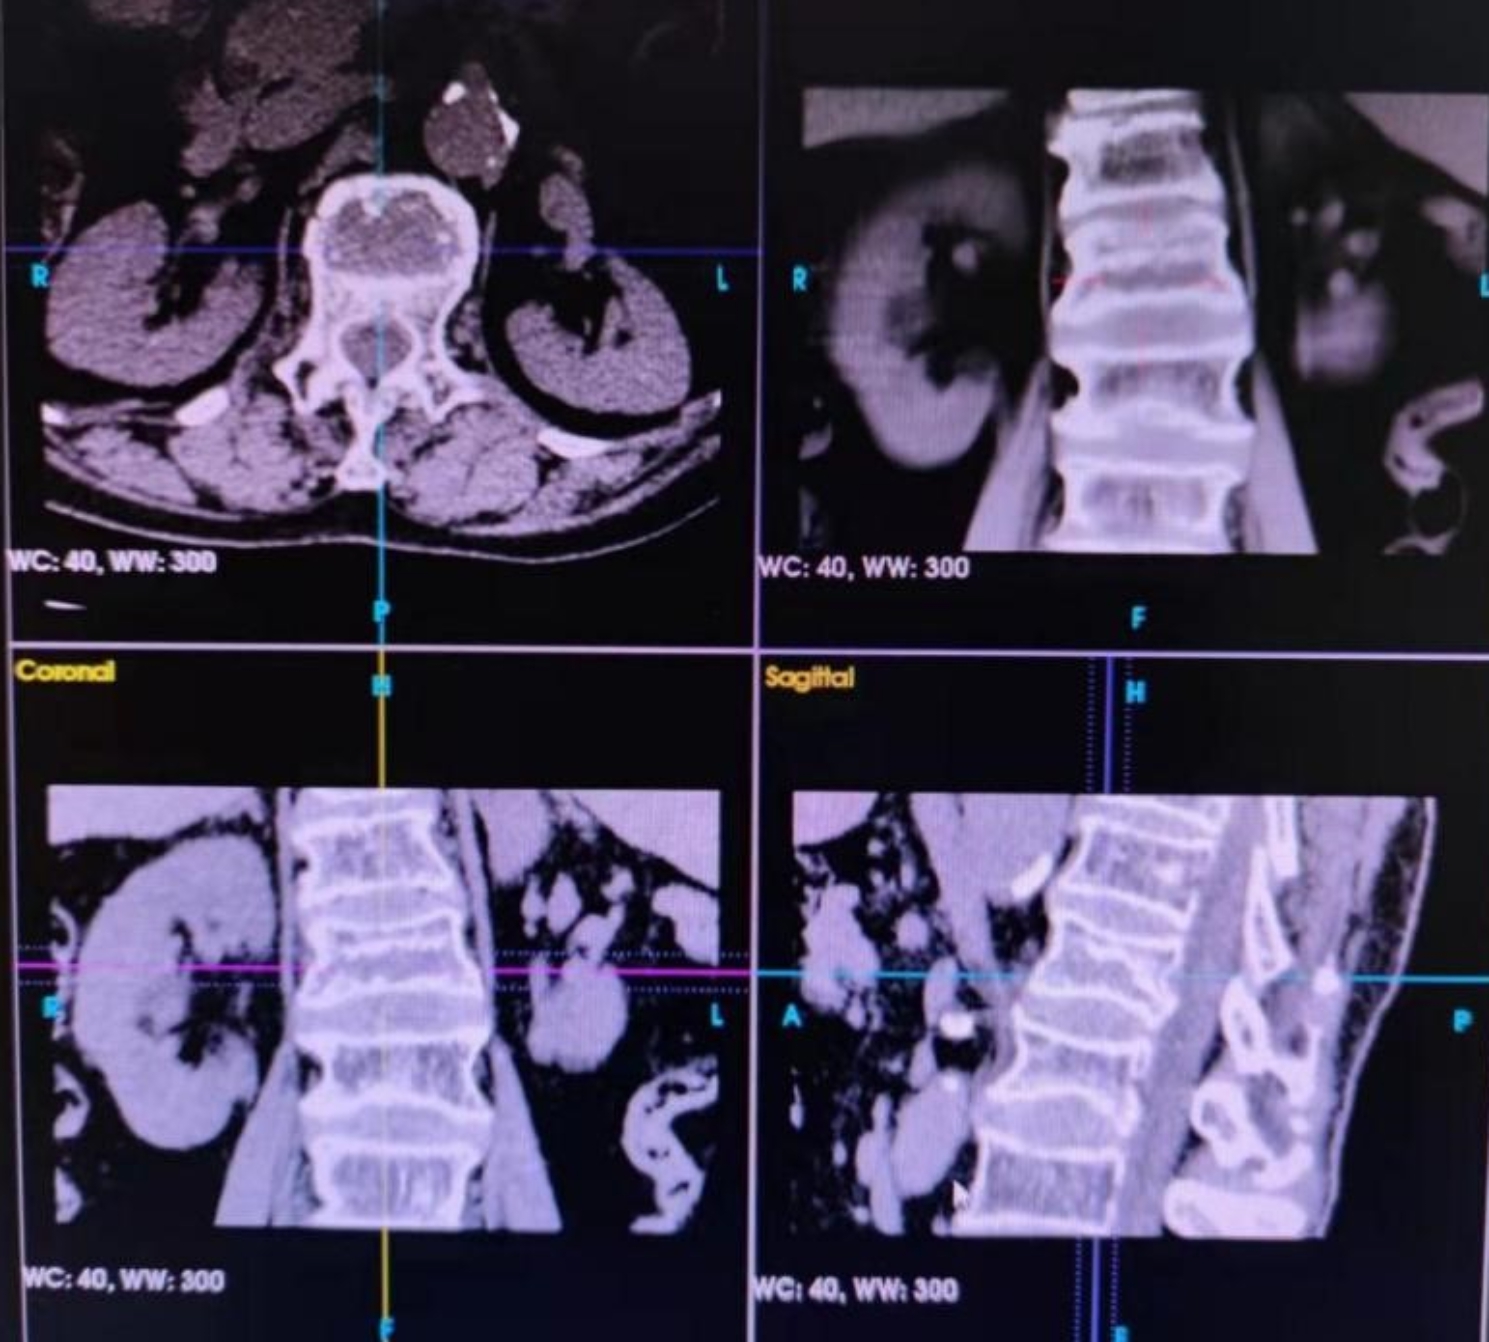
CT of a lumbar spine indicates an intact posterior wall of the lumbar 1 vertebra
